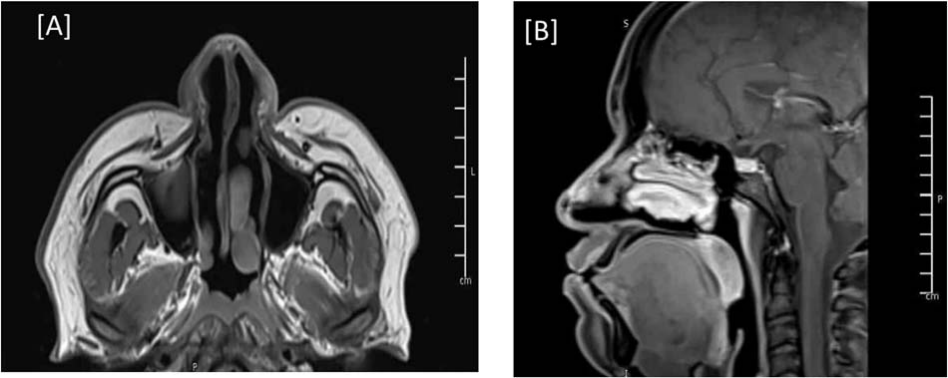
MRI of the head, and paranasal sinuses (post-op). (A, B) Axial and Mid-sagittal T1 sequence showing total resection of the external nasal mass lesion with a heterogenous enhancement seen likely postoperative changes. No obvious residual mass lesions can be seen.
